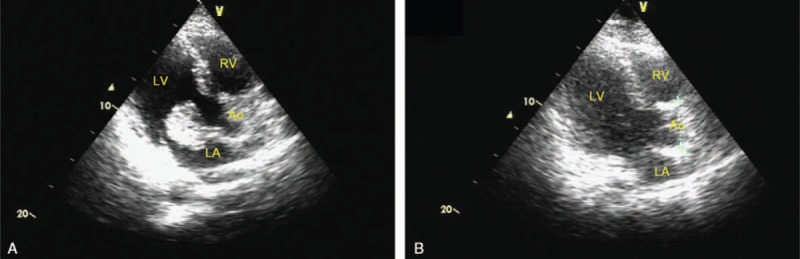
Left ventricular long axis view of TTE before and after the surgery. (A) A 43 mm × 28 mm echo mass attached on the anterior mitral leaflet; (B) the tumor was resected. TTE = transthoracic echocardiography.
